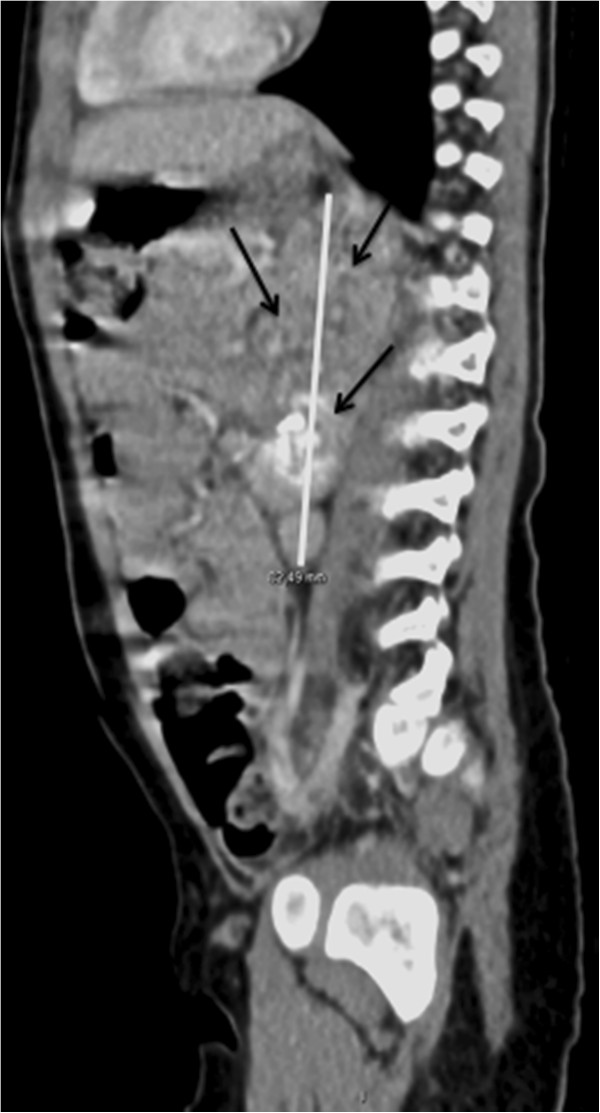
Thoracic- abdominal CT scan revealing a 83 mm*43 mm*42 mm retroperitoneal calcified heterogeneous mass (White Thick Bar) located on the left adrenal gland and perirenal region evocative of neuroblastoma (Black Arrows).
